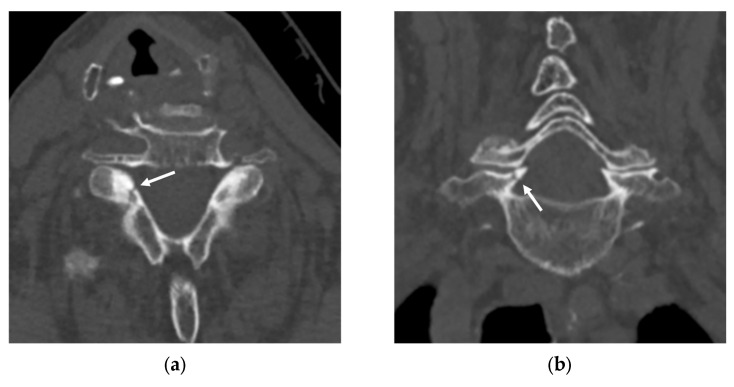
Non-enhanced CT images in an 80-year-old female with an acute nondisplaced fracture of the right superior articular process of C7. The first radiologist classified the case as “negative”, whereas the second and third radiologists identified the fracture, representing a discrepant case between the first two radiologists. The AI application classified the case as “negative”. Axial (a) and coronal (b) CT images demonstrate an acute nondisplaced fracture of the right C7 superior articular process (arrows).
